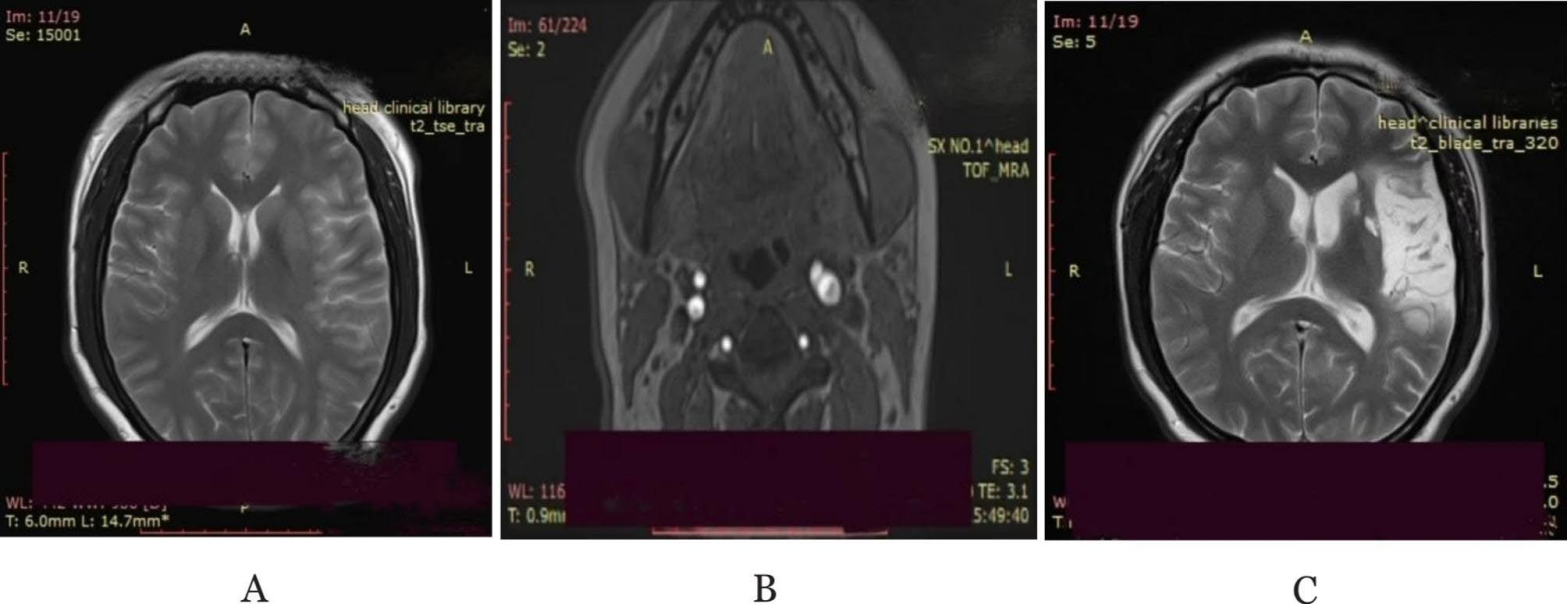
A: MRI showed hyperacute cerebral infarction in the left frontotemporal island parietal lobe; B: High-resolution magnetic resonance vessel wall imaging (HR-VWI) showed left ICA dissection; C: MRI showed no new infarctions than Figure A
